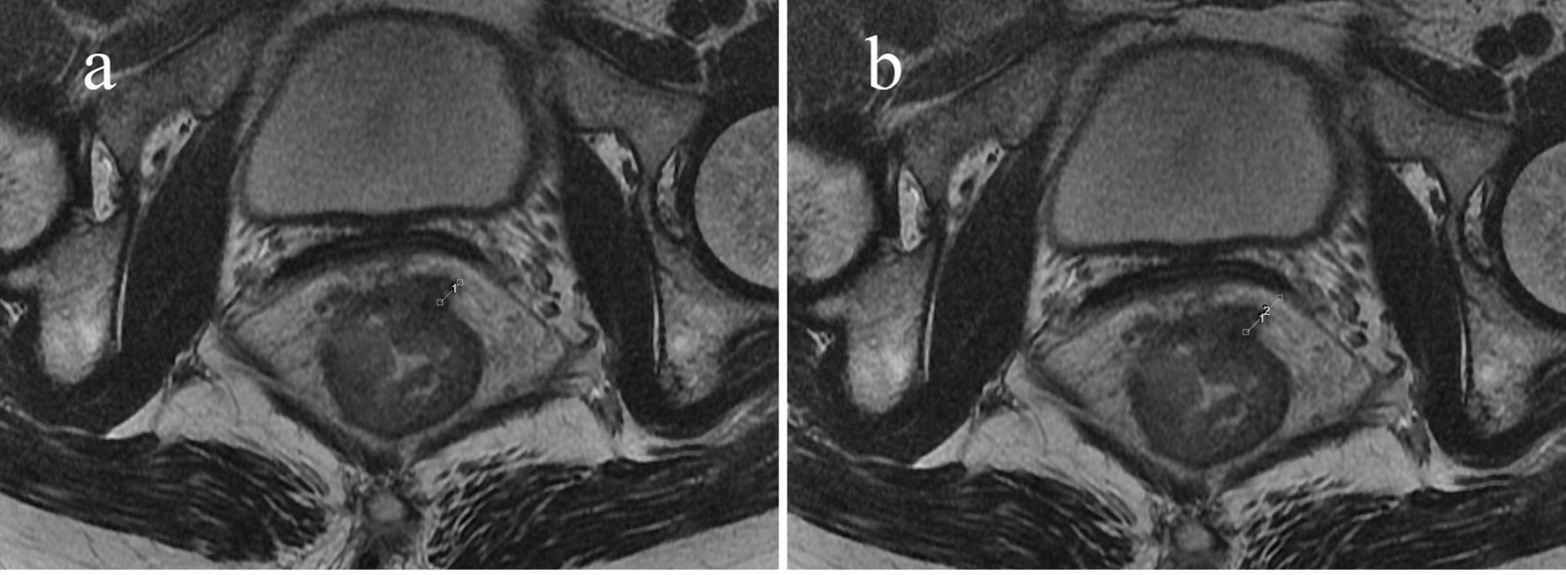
Sixty-three-year-old woman with an mr-T3N0 rectal adenocarcinoma 5 cm from the anal verge. Pretreatment rectal MRI (A, B) before NCRT. The EMD was 6.1 mm (A), TM was 10.5 mm (B), and the calculated PTI was 58.1%. The rectal cancer had slightly regressed after NCRT. The postoperative staging confirmed by pathology was T3N0, and the tumor response was non-pCR.
